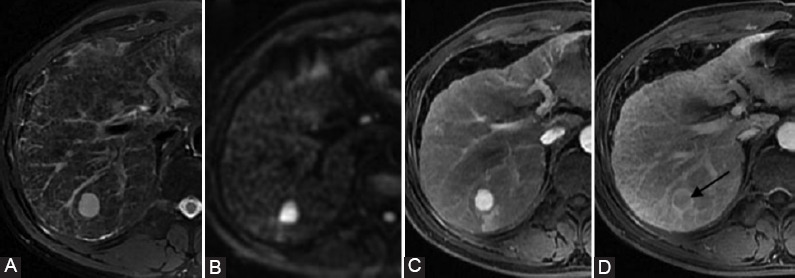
LR-5 intrahepatic cholangiocarcinoma (ICCA). A surgically confirmed ICCA in a 46-year-old male with HBV infection. (A) T2WI-FS image, (B) diffusion-weighted imaging (DWI) image (b = 1000 sec/mm), (C) late arterial, and (D) delayed phase images. The tumor showed high signal intensity on T2WI-FS and DWI images arterial phase hyperenhancement (not rim) during the late arterial phase and washout (not peripheral) during the delayed phase. (D) In delayed phase, the tumor showed enhancing capsule appearance (arrow).
